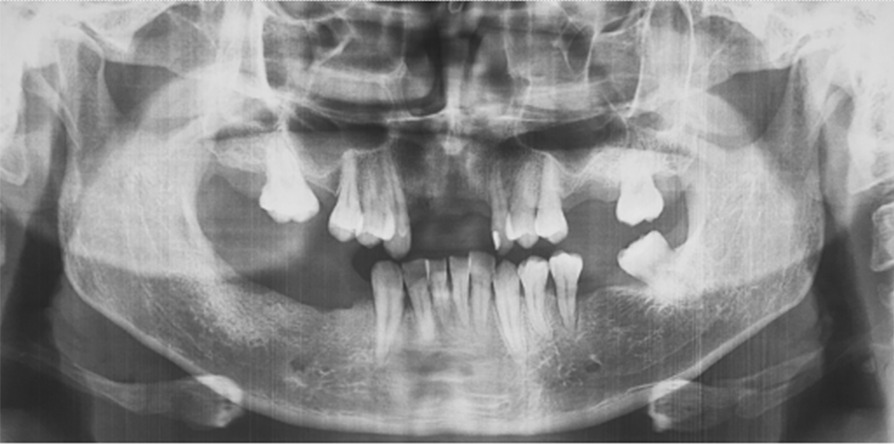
Postoperative panoramic radiograph of the patient, revealing both sizes of the mandible with symmetric shape, proportion, and density
